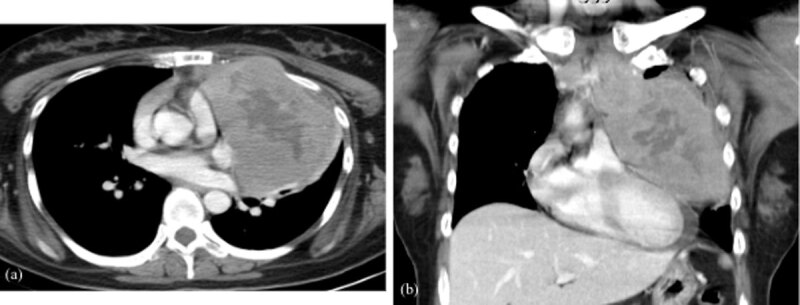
Post-contrast chest CT scan axial (a) and coronal (b) views: A large heterogeneous enhancing anterior mediastinal mass with intrinsic necrosis.
